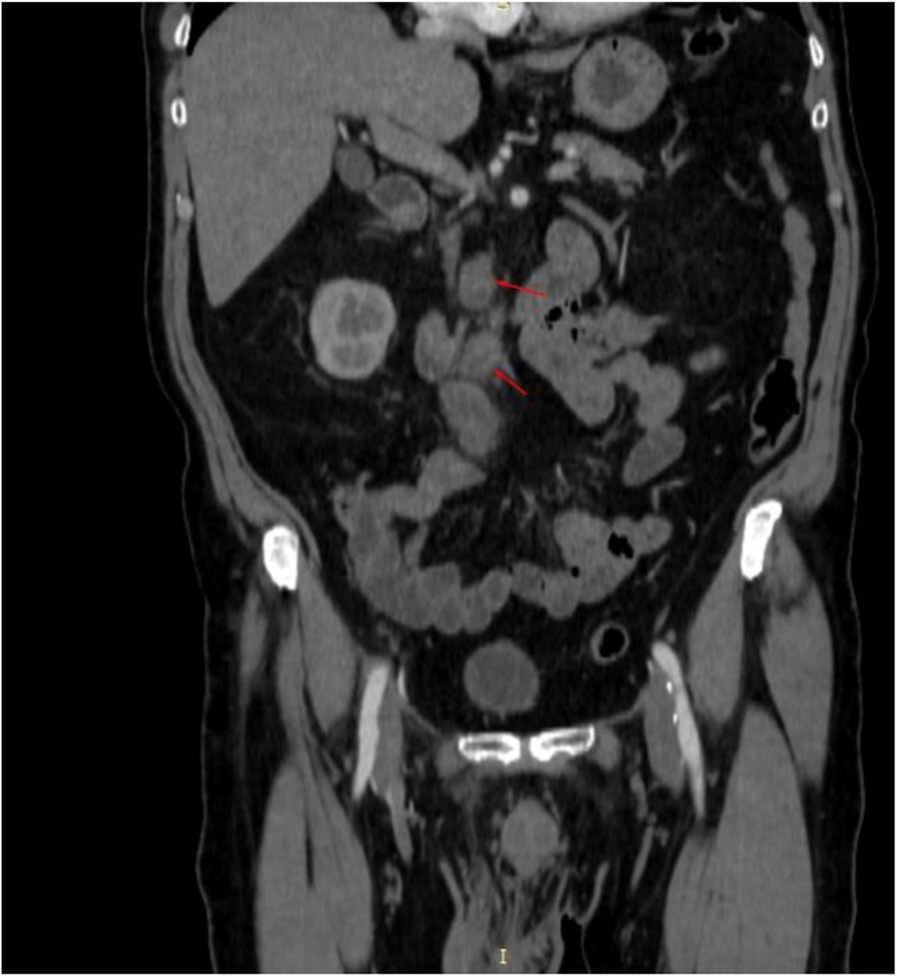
Infrarenal paraaortic masses enlarged to 21 × 25 mm, next to aortic bifurcation masses enlarged to 15 mm in computed tomography coronal view
